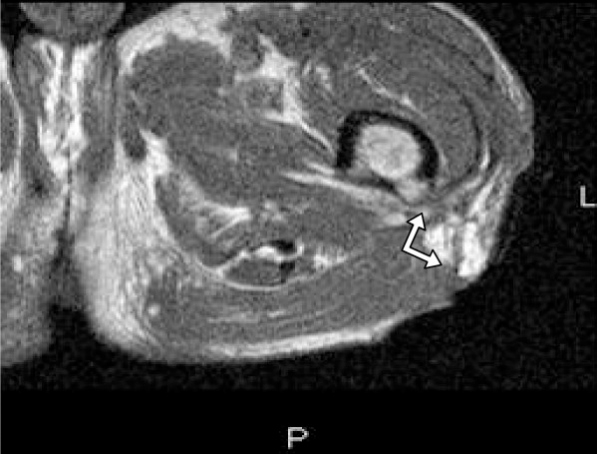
MRI of the left thigh showing a sinus tract (↔) from the proximal femur.
